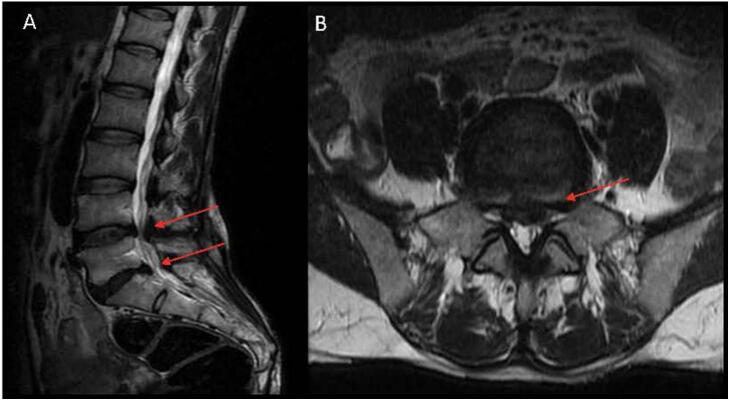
Patient 2: Preoperative MRI. (A) Sagittal T2-weighted MRI showing central stenosis at L4–5 and L5-S1, secondary to degenerative disc disease. (B) Axial T2-weighted image showing significant central/paracentral and lateral recess stenosis at L5-S1.
